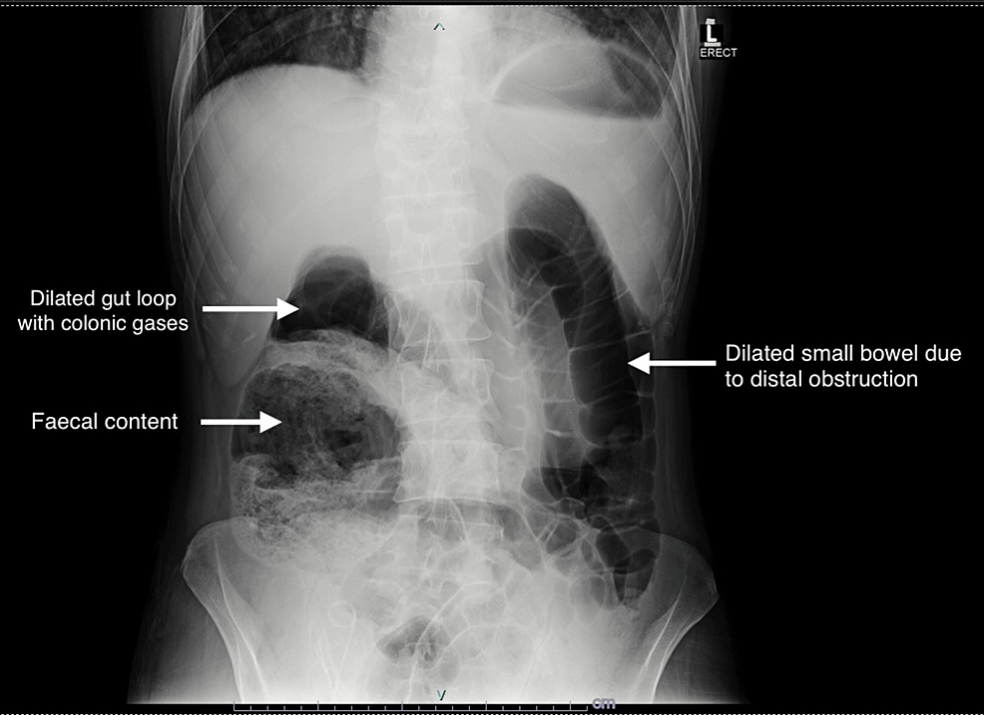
Abdominal X-ray showing dilated gut loops with colonic gases and fecal content on the right side along with dilated small bowel due to distal obstruction on the left side.
